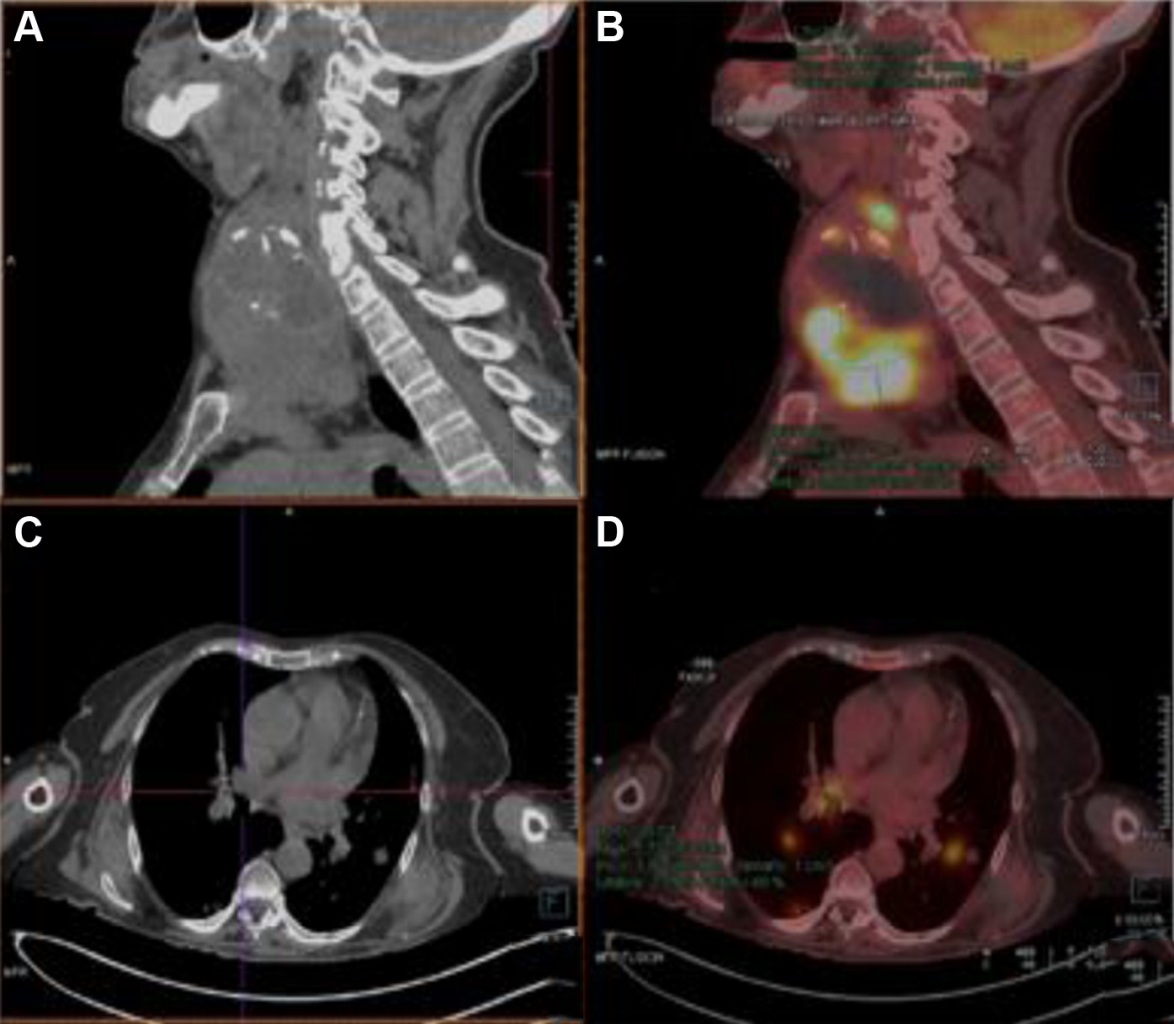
A, B, Sagittal computed tomography and positron emission tomography scans showing a multinodular goiter and a hyperintensity focus (tumor). C, D, Cross-section computed tomography and positron emission tomography scans showing an intracardiac thrombus and a hyperintensity focus (tumor).
